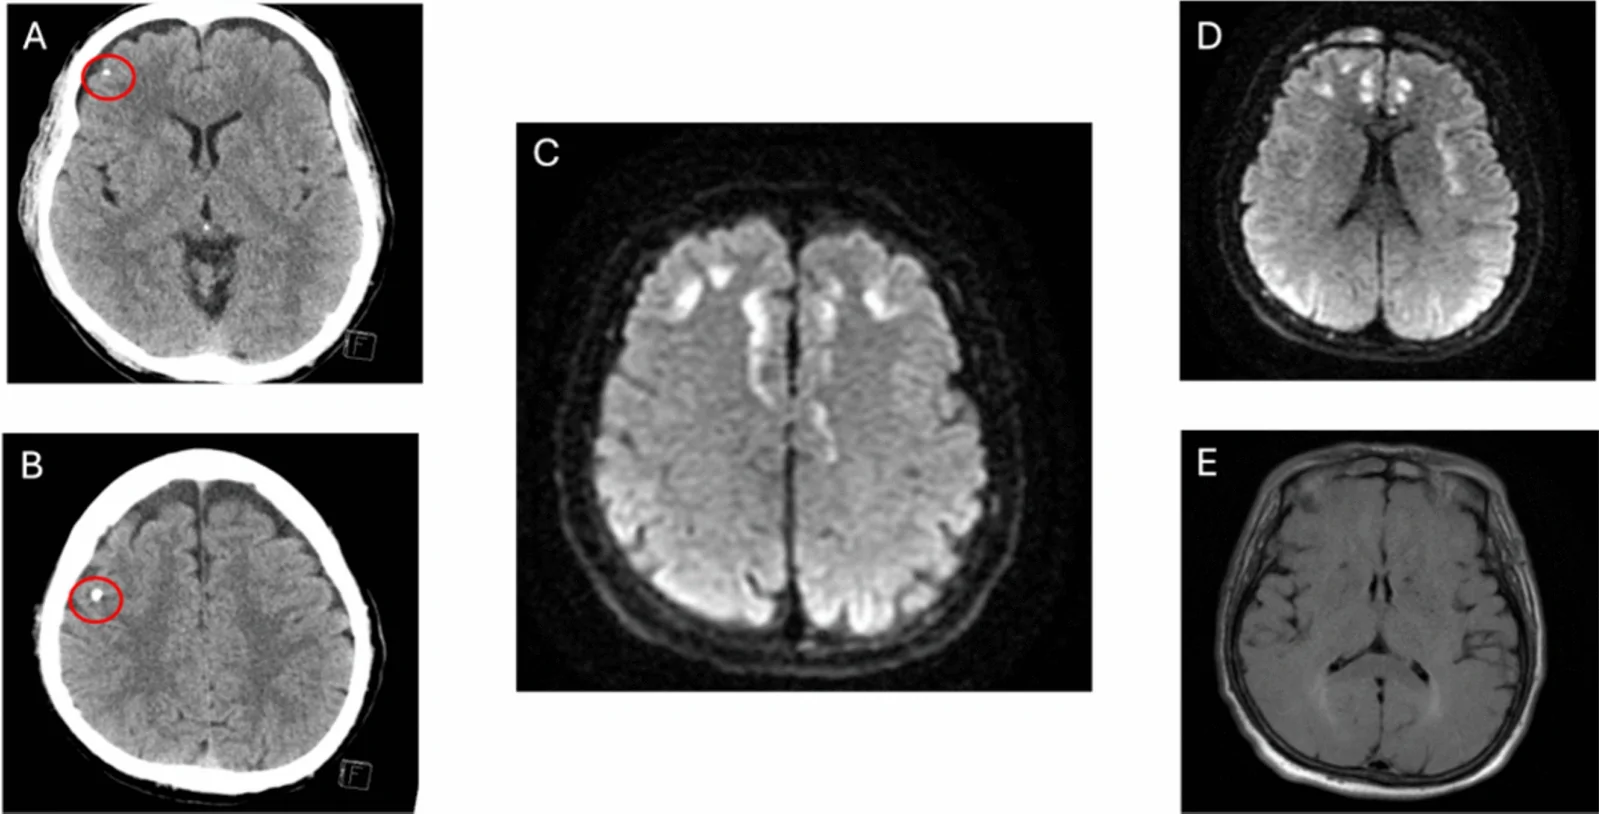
Patient’s brain imaging. A and B Computed Tomography (CT) images revealing calcifications depicting old neurocysticercosis (indicated by red circles). C DWI image showing hyperintense enhancements in the bilateral frontal lobe cortices with punctate microhemorrhages. D Diff usion-Weighted Imaging (DWI) image revealing hyperintense left insular enhancement. E T2-weighted Fluid-Attenuated Inversion Recovery (T2-FLAIR) image showing no thalamic involvement. CT: Computed Tomography; DWI: Diffusion-Weighted Imaging; T2-FLAIR: T2-weighted Fluid-Attenuated Inversion Recovery
